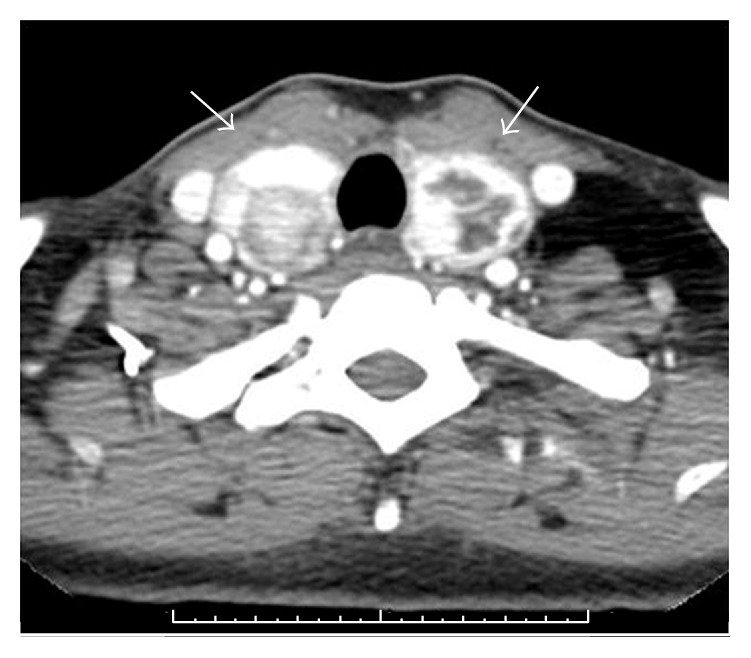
Axial postcontrast CT image through the thyroid gland demonstrates enlarged thyroid lobes that contain heterogeneously enhancing nodules bilaterally.
